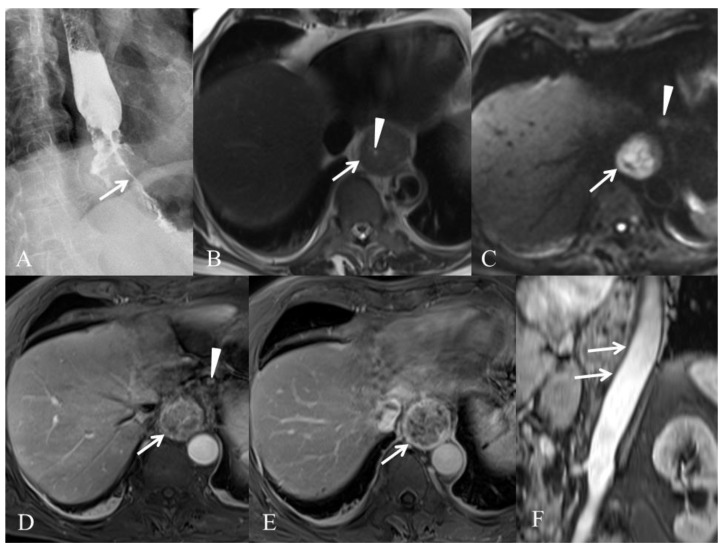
Initial staging for a 77-year-old man with T3N1M0 esophageal adenocarcinoma. (A) Esophagram shows esophageal tumor of the lower third of the esophagus responsible for marked luminal narrowing (arrow). (B) T2-weighted HASTE MR image in the axial plane shows esophageal tumor (arrow) with luminal narrowing (arrowhead). (C) Diffusion-weighted MR image in the axial plane obtained with high b value (b = 800 s/mm^2^) shows restricted diffusion (arrow) consistent with malignant esophageal tumor. Additional hyperintense lymph node is present (arrowhead). (D) T1-weighted VIBE image in the axial plane obtained 30 s after intravenous administration of a gadolinium-based contrast agent (gadoterate meglumine, Dotarem^®^, Guerbet, Villepinte, France) shows heterogeneous esophageal tumor (arrow) and enhancing lymph node (arrowhead). (E) T1-weighted VIBE image in the axial plane obtained 60 s after intravenous administration of a gadolinium-based contrast agent (gadoterate meglumine, Dotarem^®^, Guerbet) shows that the tumor is well delineated without spreading outside the adventitia (arrow). (F) MR angiography image in the oblique plane shows intact interface (arrows) between esophageal tumor and aorta.
